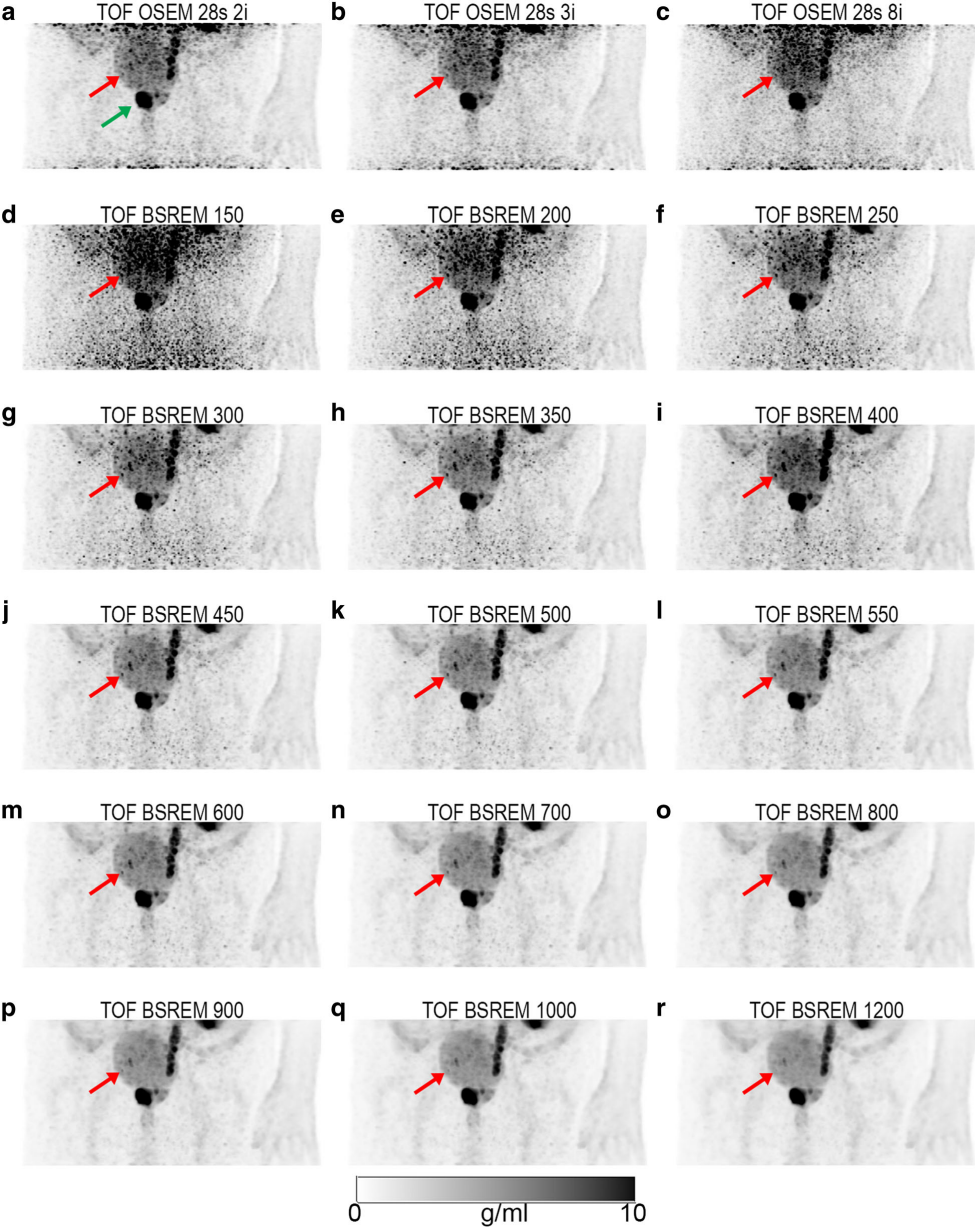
Example dataset showing coronal PET maximum intensity projections (MIP) of the pelvic frame. PSMA accumulation is seen in the left ureter; besides the high activity in the primary tumor (green arrow), a small lymph node can be detected (red arrow). The reconstructions are TOF OSEM with 28 subsets and 2 (a), 3 (b), and 8 (c) iterations; TOF BSREM with regularization parameter *β* = 150 (d), 200 (e), 250 (f), 300 (g), 350 (h), 400 (i), 450 (j), 500 (k), 550 (l), 600 (m), 700 (n), 800 (o), 900 (p), 1000 (q), and 1200 (r). All images have SUV scale 0–10 g/ml. SUV standardized uptake value, TOF time-of-flight, OSEM ordered subset expectation maximization, BSREM block sequential regularized expectation maximization
